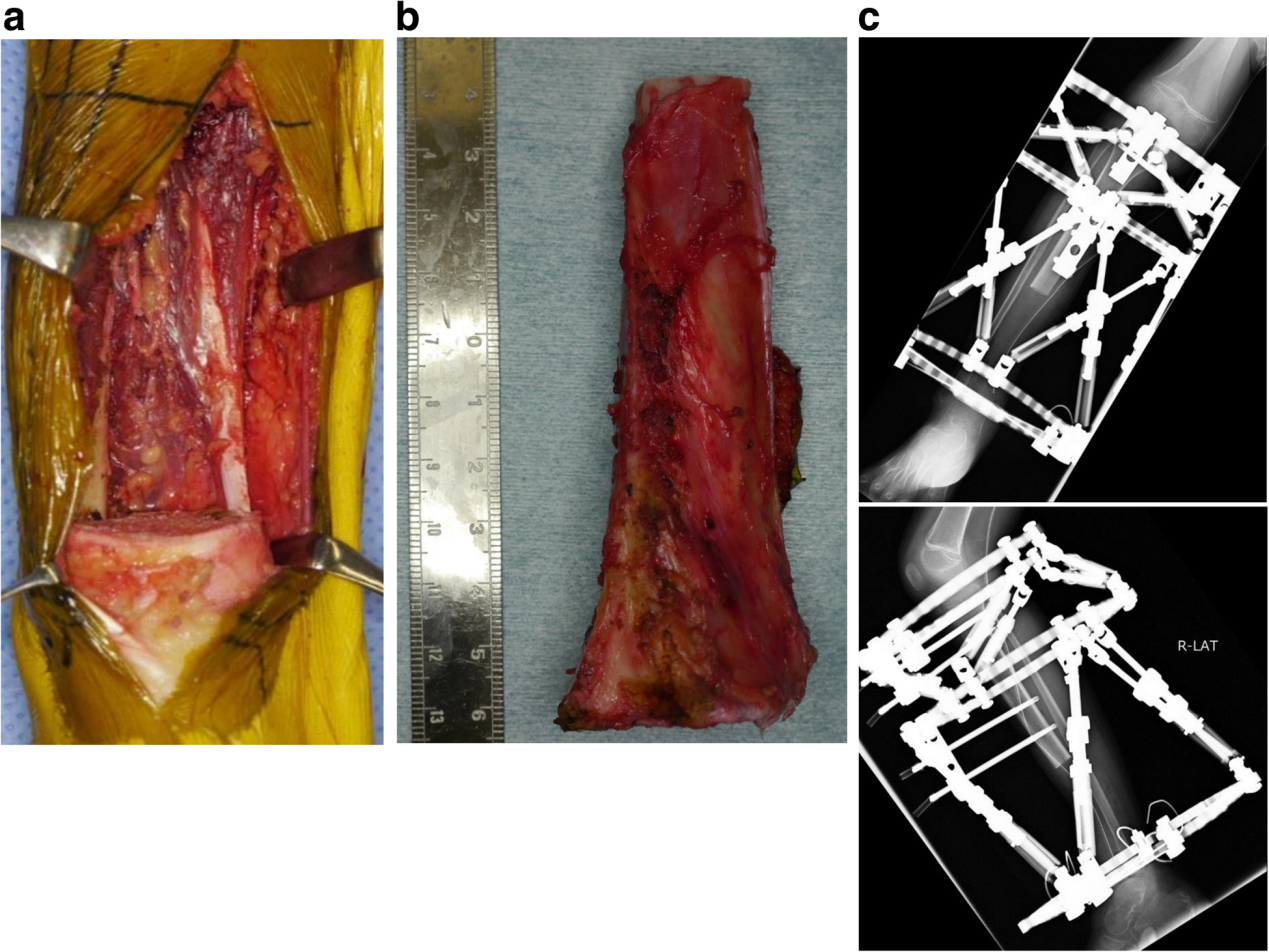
a After wide excision, no tumor remained. b The excised bone fragment was about 95 mm. c X-ray following reconstruction, showing Taylor Spatial Frame for bone transportation
